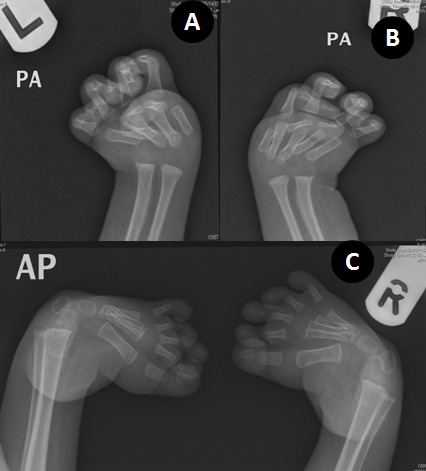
Figure 3: (A) 4D reconstructed colored CT image of face showing round forehead, prominent cheeks, broad nasal bridge, long philtrum, small nose, hypoplastic nostrils, and small mouth with whistling appearance. (B) 4D reconstructed bony image of the skull / face showing prominent forehead, patent anterior fontanel, non fused coronal suture, fused sagittal suture, hypotelorism with large orbits, long philtrum, and abnormal dentition. (C) Plain X ray chest and abdomen AP view showing hypoplastic cervical vertebral bodies, scoliosis of dorsal spine, wide ribs with narrow vertebral ends, normal lung inflation and normal bowel gas shadows.
